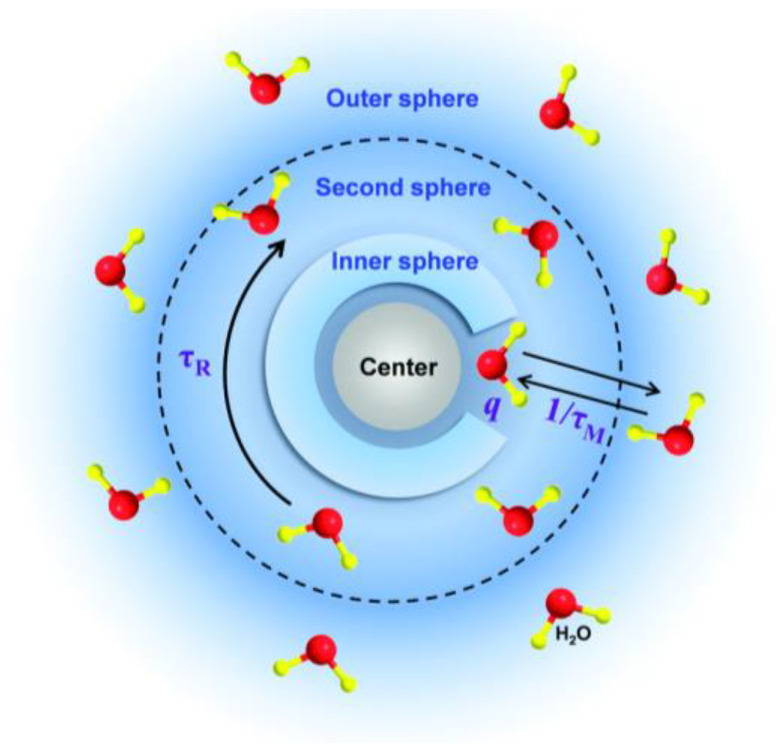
The inner sphere and outer sphere can influence the relaxation rates of MRI CAs [132]. Reprinted with permission from [132].
